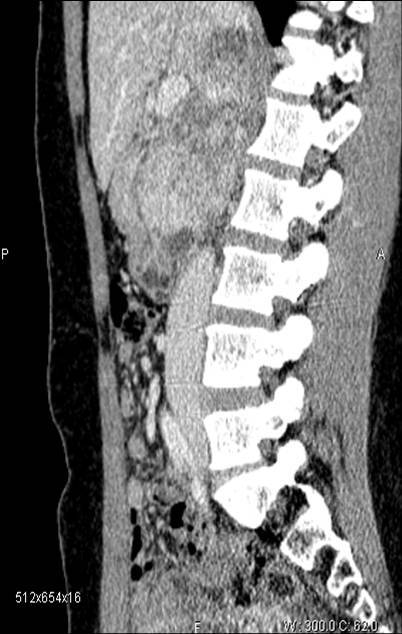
CT scan before surgery—patient 12. Video clip. Surgical procedure of mass resection with cava reconstruction. Patient 12 To view this video click here: http://www.ecancermedicalscience.com/view-article.asp?doi=10.3332/ecancer.2009.158
